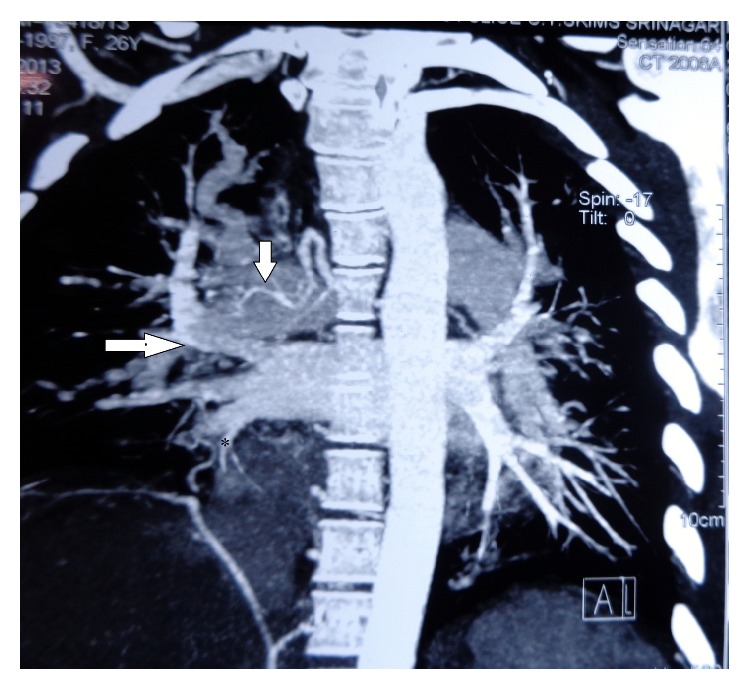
CT pulmonary angiography showing filling defects suggestive of thrombi in the pulmonary vessels (horizontal arrow). Pruning of pulmonary vessels is seen (asterisk). Also collateral vessels have started forming (vertical arrow).
